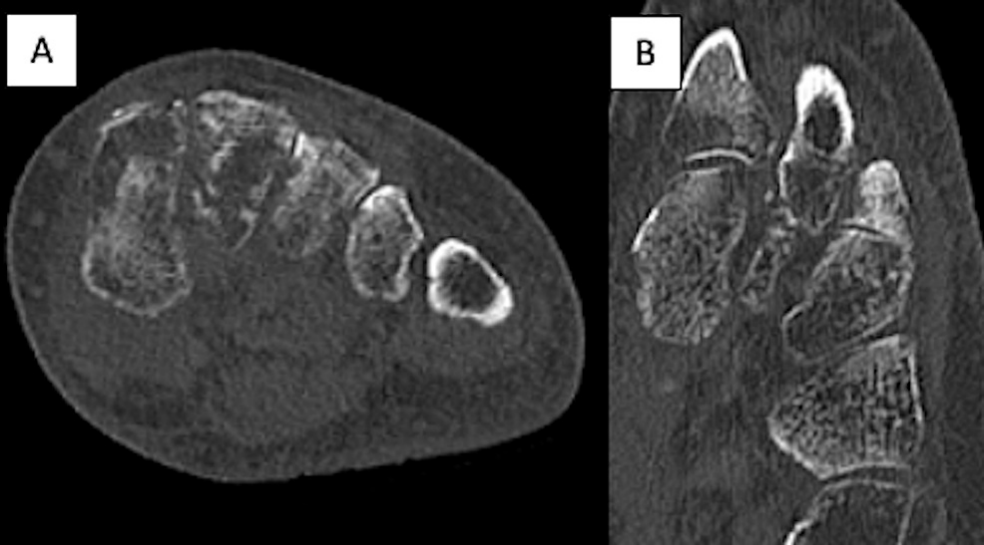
Left foot CT. Images of the left foot demonstrating a subacute Lisfranc injury with a nondisplaced avulsion fracture to the base of the second metatarsal. CT = computed tomography
